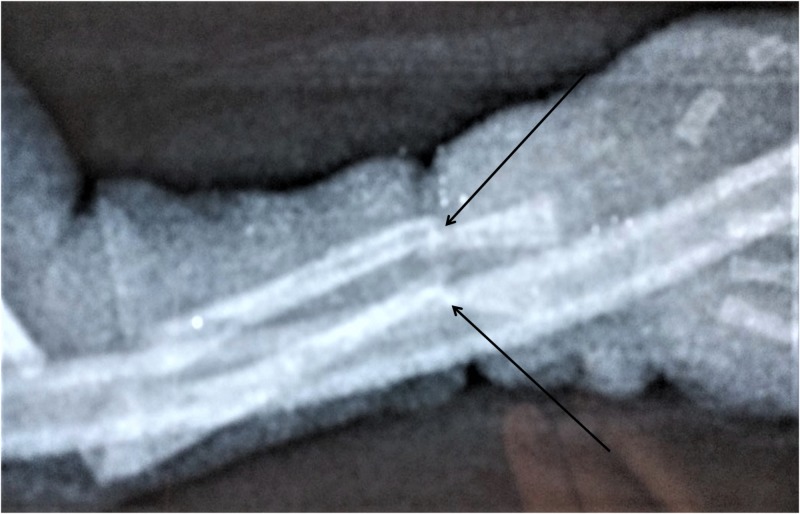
Radiograph of the fracture site after reduction.
